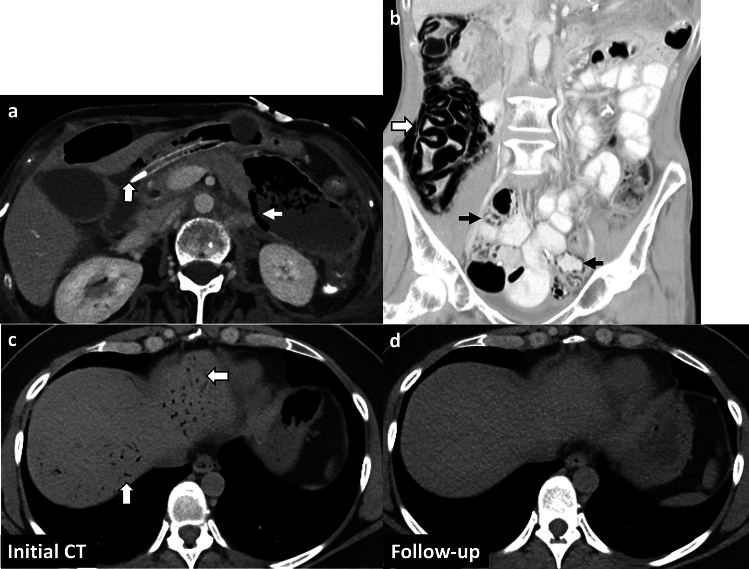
Benign pneumatosis intestinalis. a Pneumatosis intestinalis associated with feeding jejunostomy. A 54-year-old with breast cancer and disseminated metastases on feeding jejunostomy (white block arrow). The jejunal loop is dilated with pneumatosis (white arrow), which was unchanged on multiple CTs over 3 months. Serum lactate was normal at all time points, confirming benign pneumatosis intestinalis. b Pneumatosis intestinalis and coli associated with molecular targeted therapy. A 56-year-old with renal cancer on cabozantinib therapy. There is benign pneumatosis intestinalis (black arrows) and pneumatosis coli (white block arrow). Findings were unchanged over 6 months. c, d Portal venous gas associated with accidental hydrogen peroxide ingestion. A 39-year-old with epigastric pain, vomiting, and foaming at the mouth. Initial CT (c) shows portal venous gas (white block arrow, c) concerning for mesenteric ischemia. Serum WBC and serum lactate were normal. The patient was treated with hyperbaric oxygen therapy, and findings completely resolved on follow-up CT (d)
